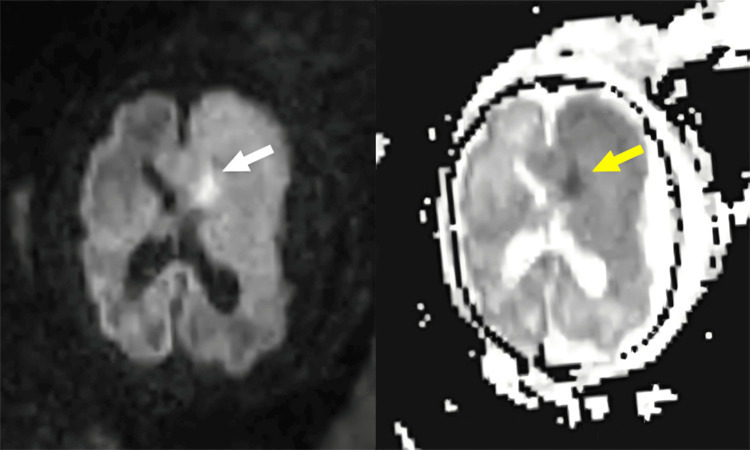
Fetal magnetic resonance imaging, diffusion sequences (DWI, ADC). Tissue diffusion restriction in the left frontal region, indicated by hyperintensity in DWI (white arrow) and hypointensity in ADC (yellow arrow). DWI: diffusion-weighted imaging, ADC: apparent diffusion coefficient
